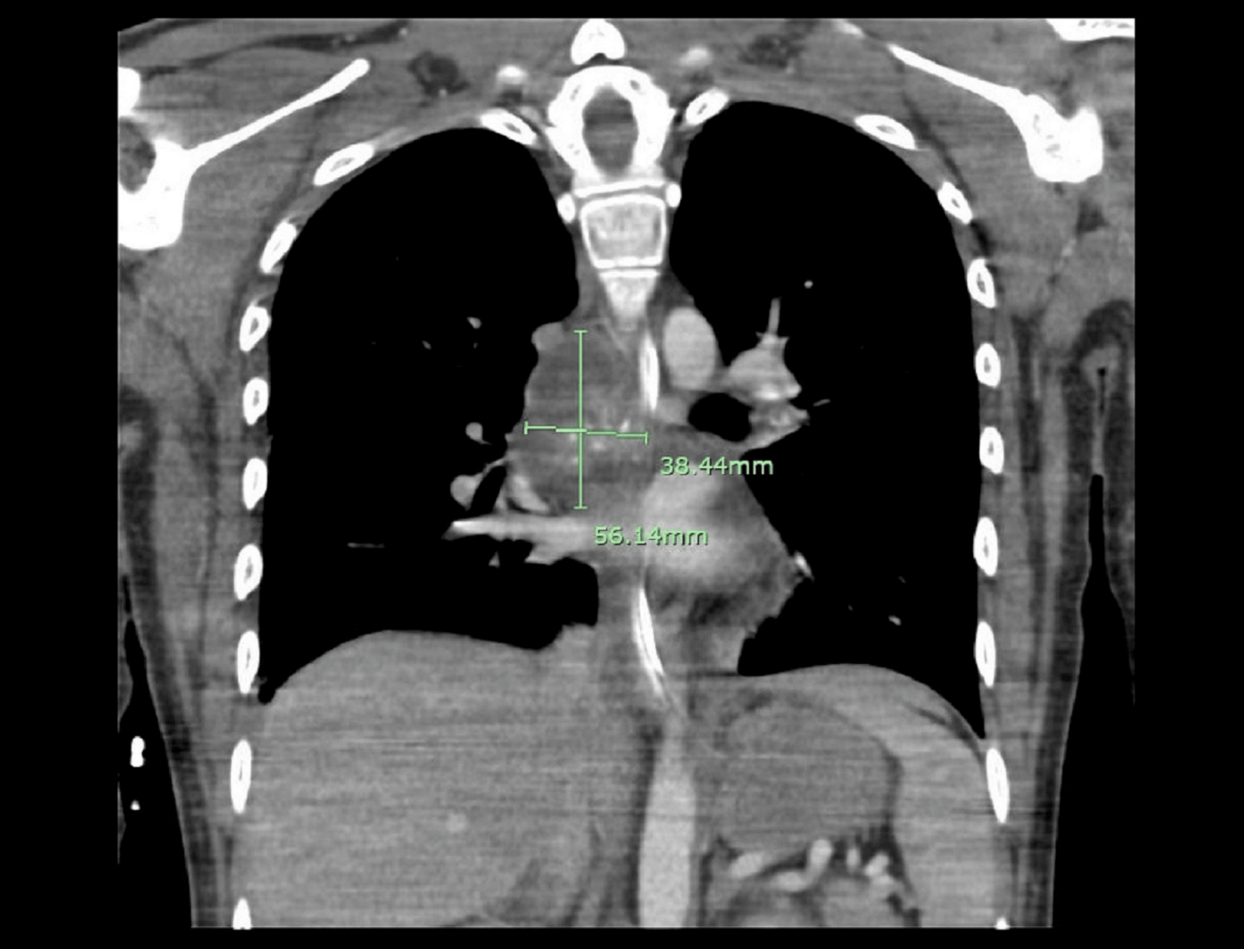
CT of the thorax with contrast. Coronal CT of the thorax with contrast demonstrating a partially calcified posterior mediastinal lesion measuring 4.7×2.6×4.7 cm. CT: computed tomography
